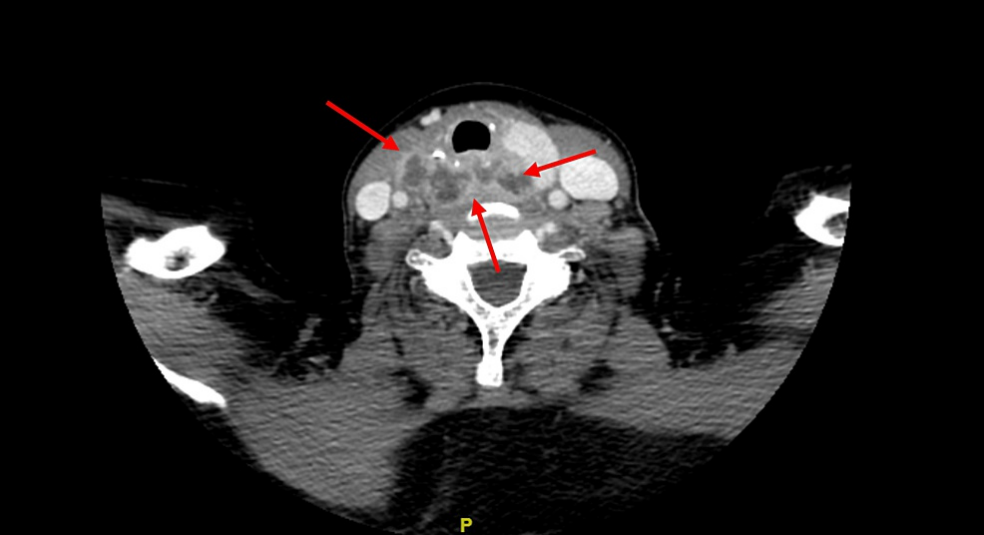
CT scan of the neck with IV contrast demonstrates a collection of hypodense rim-enhancing lesions interposed between the esophagus and trachea (red arrows).
